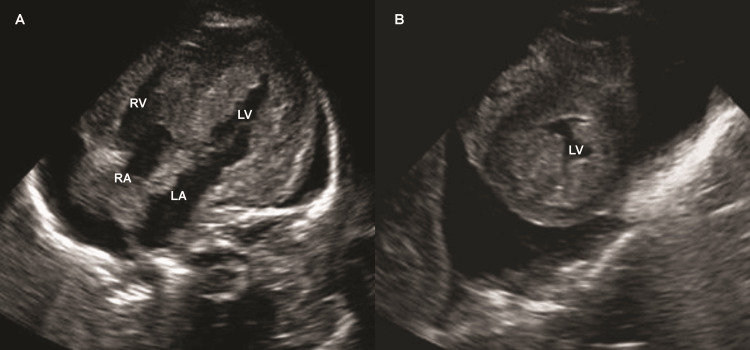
Transthoracic echocardiography Echocardiography in the apical four-chamber (A) and short-axis (B) views revealed severe ventricular hypertrophy and pericardial effusion. RV: right ventricle; RA: right atrium; LV: left ventricle; LA: left atrium
